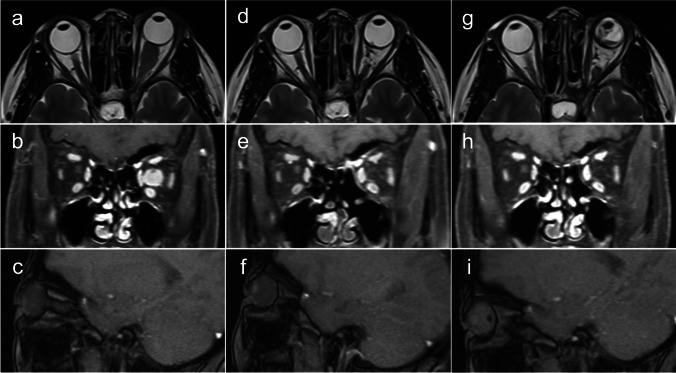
Case 11 presentation. MRI showing a left-sided ONSM underwent ETA. a–c At 1-day preoperative axial, coronal, and sagittal T1/T2-weighted MR images; d–f at 6-month postoperative axial, coronal, and sagittal T1/T2-weighted MR images; g–i at 34-month postoperative axial, coronal, and sagittal T1/T2-weighted MR images
